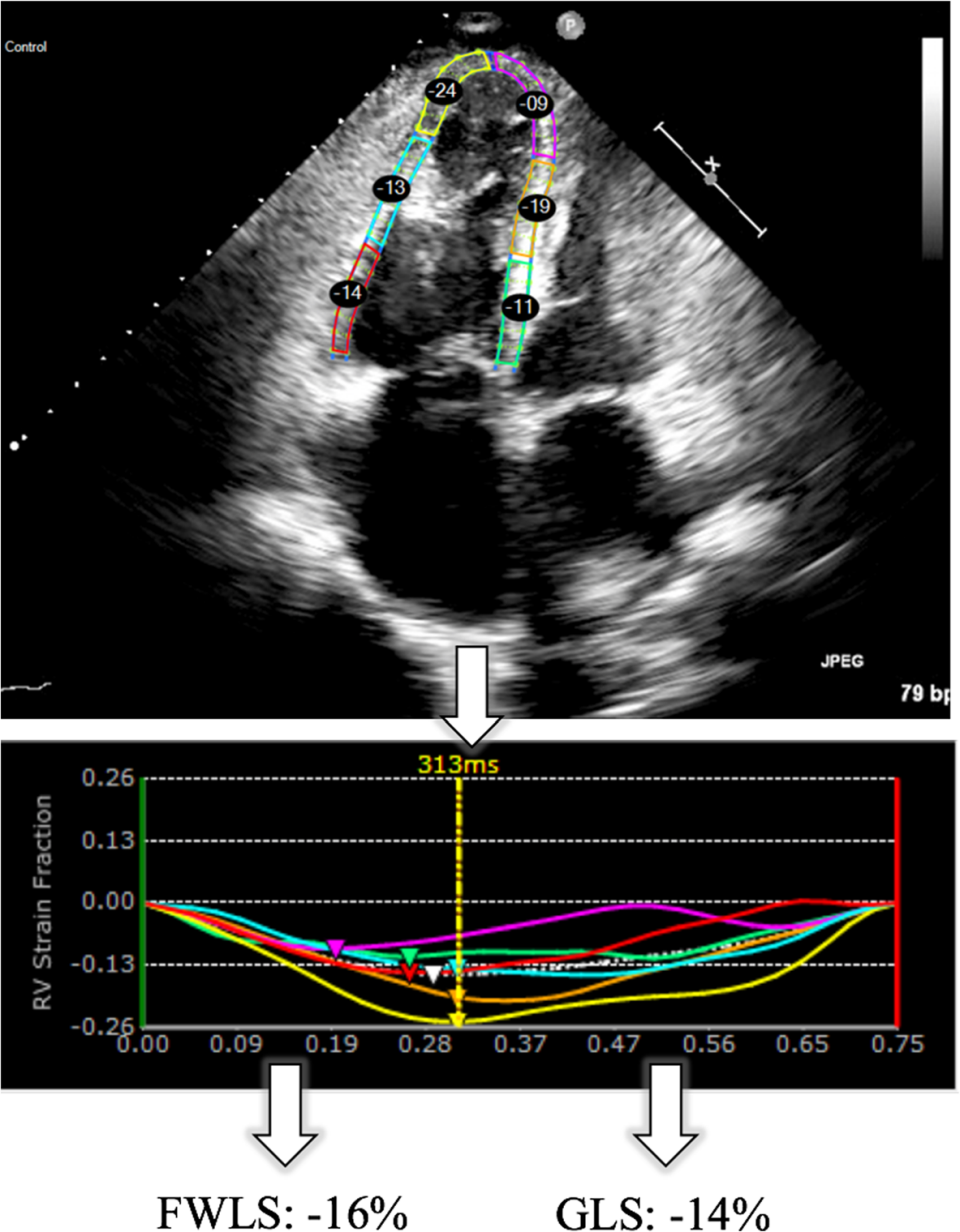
Representative speckle tracking echocardiography (STE)-image, strain curves and end-systolic strain values of a patient with pulmonary artery hypertension and right-sided heart failure (LVEF:56%, RVEF:28%). LVEF, left ventricular ejection fraction; RVEF, right ventricular ejection fraction. GLS = global longitudinal strain, FWLS = free wall longitudinal strain
